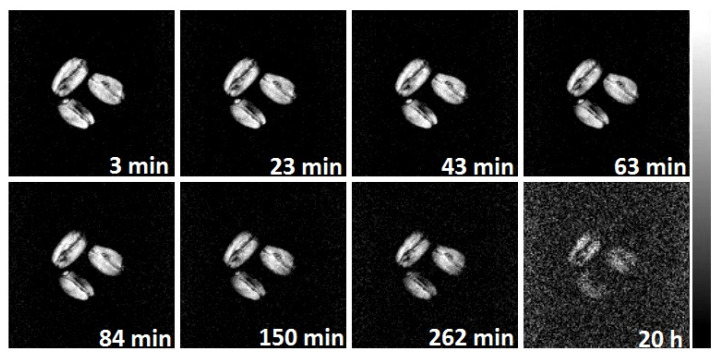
MRI images of wheat grains during sorption drying at 22 °C. The time elapsed from the beginning of the drying process is indicated. Higher signal intensity corresponds to higher moisture level.
